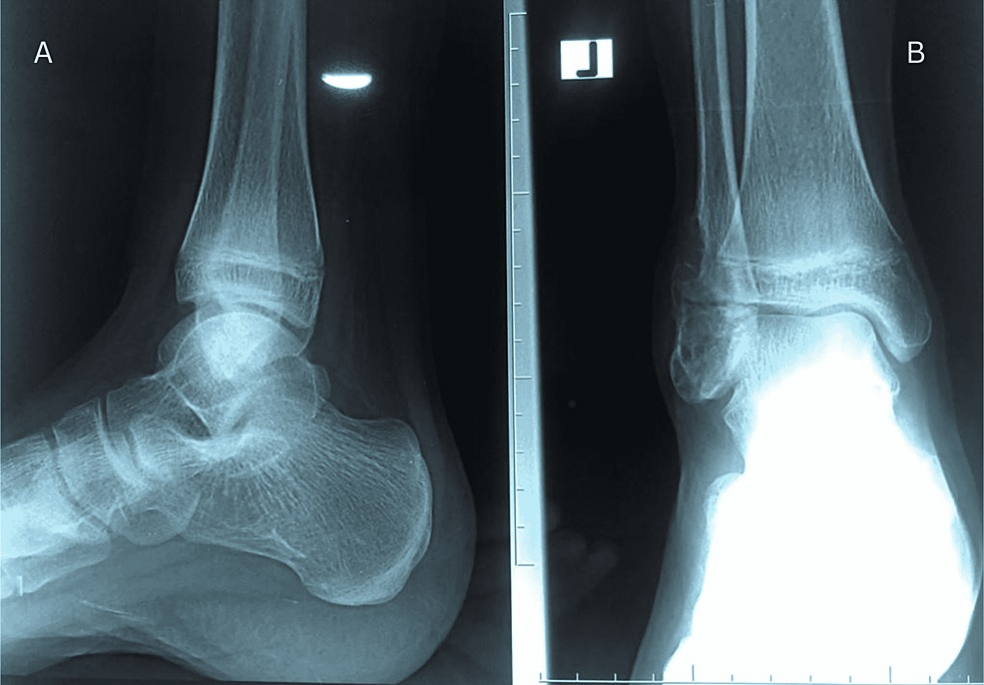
Pre-operative X-ray results. The lateral (A) and anteroposterior (B) views of the left ankle show an osteolytic balloon lesion of the lateral malleolus of the distal fibula. Septations within the tumor, a thin cortex, and a narrow zone of transition were also observed.
